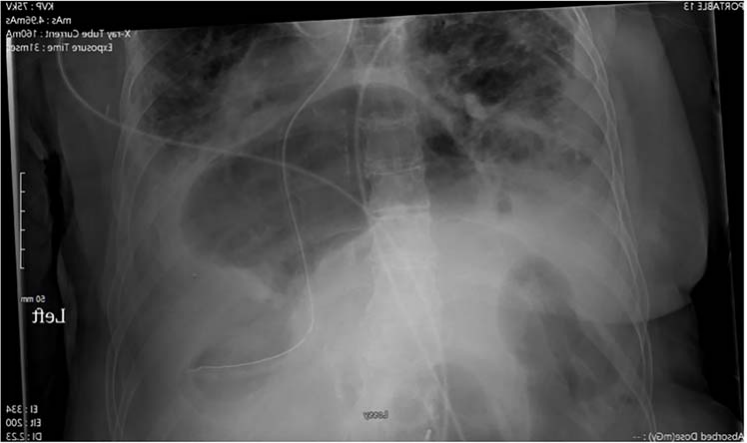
X-ray of the abdomen: anterior–posterior portable X-ray of the abdomen showing bowel gas pattern in the right upper abdomen and a large hiatal hernia with gaseous distention of the stomach.
